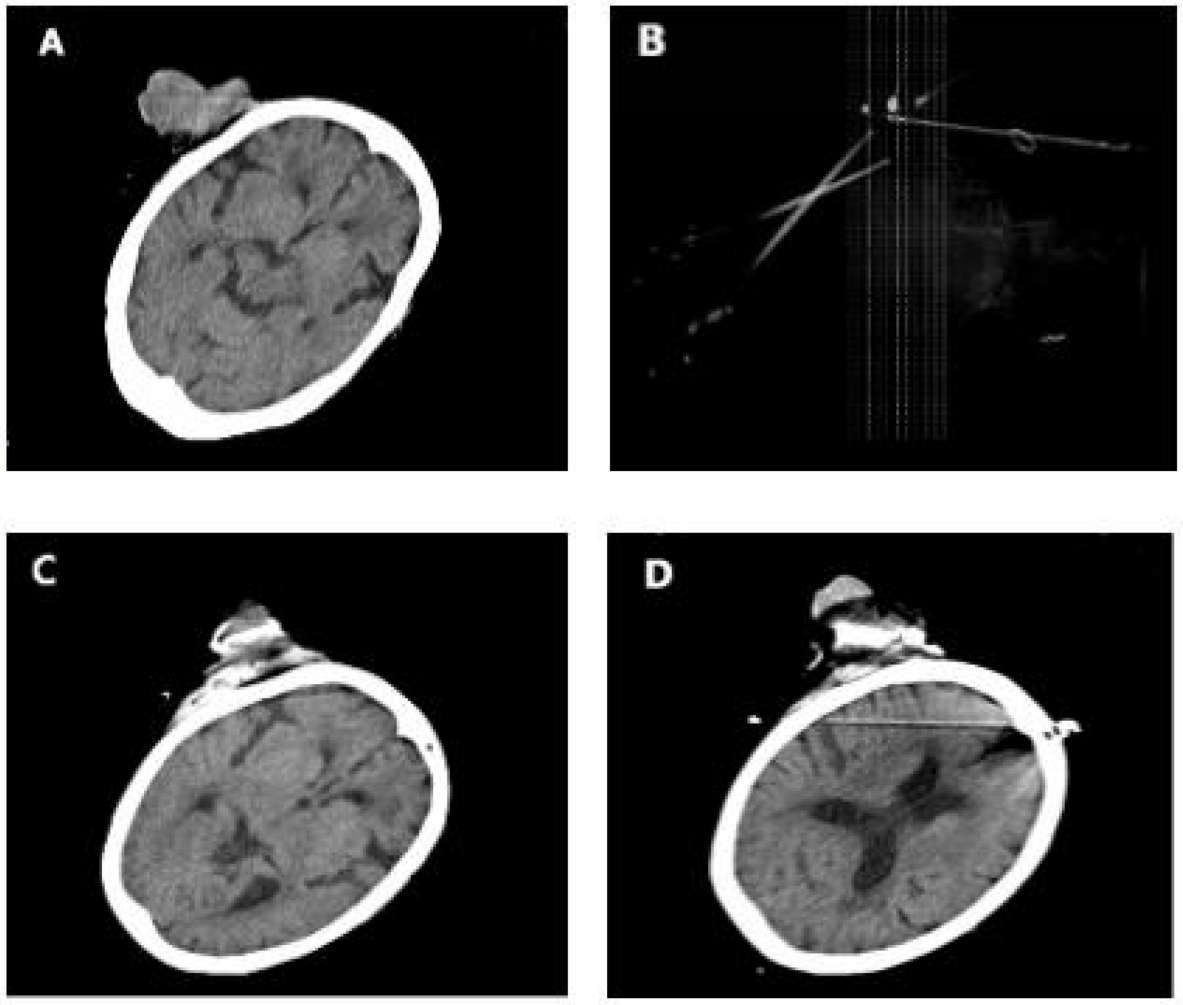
(A) Case 3 before cryotherapy. (B) Three cryoprobes were placed under CT guidance according to the size and location of the tumor. (C, D) During cryotherapy. The patients passed away due to unrelated diseases 3 years after cryotherapy.
